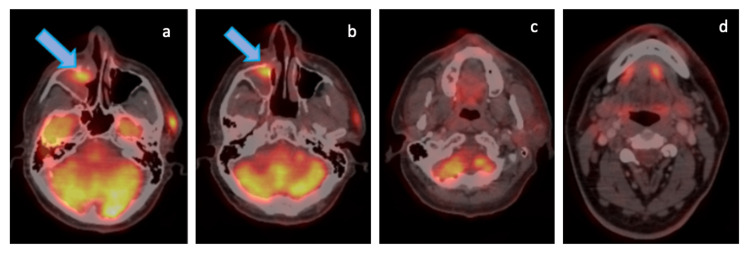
Pretreatment F-18 fluorodeoxyglucose (FDG) Positron Emission Tomography (PET)/Computed Tomography (CT) (a-d)
